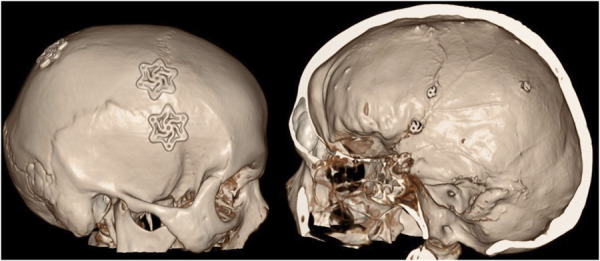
Left, A right-lateral 3D CT reconstruction of patient 1 demonstrating the position of the navigated pterional burr hole, here covered with a titanium plate. Right, A view from the inside-out perspective, displaying the inner skull surface, confirming that the burr hole was precisely positioned at the pterional MMA sulcus. Note the impressions of the MMA branches on the inner skull surface and how the standard frontal burr hole already targeted a distal branch. 3D, 3-dimensional; CT, computed tomography; MMA, middle meningeal artery.
